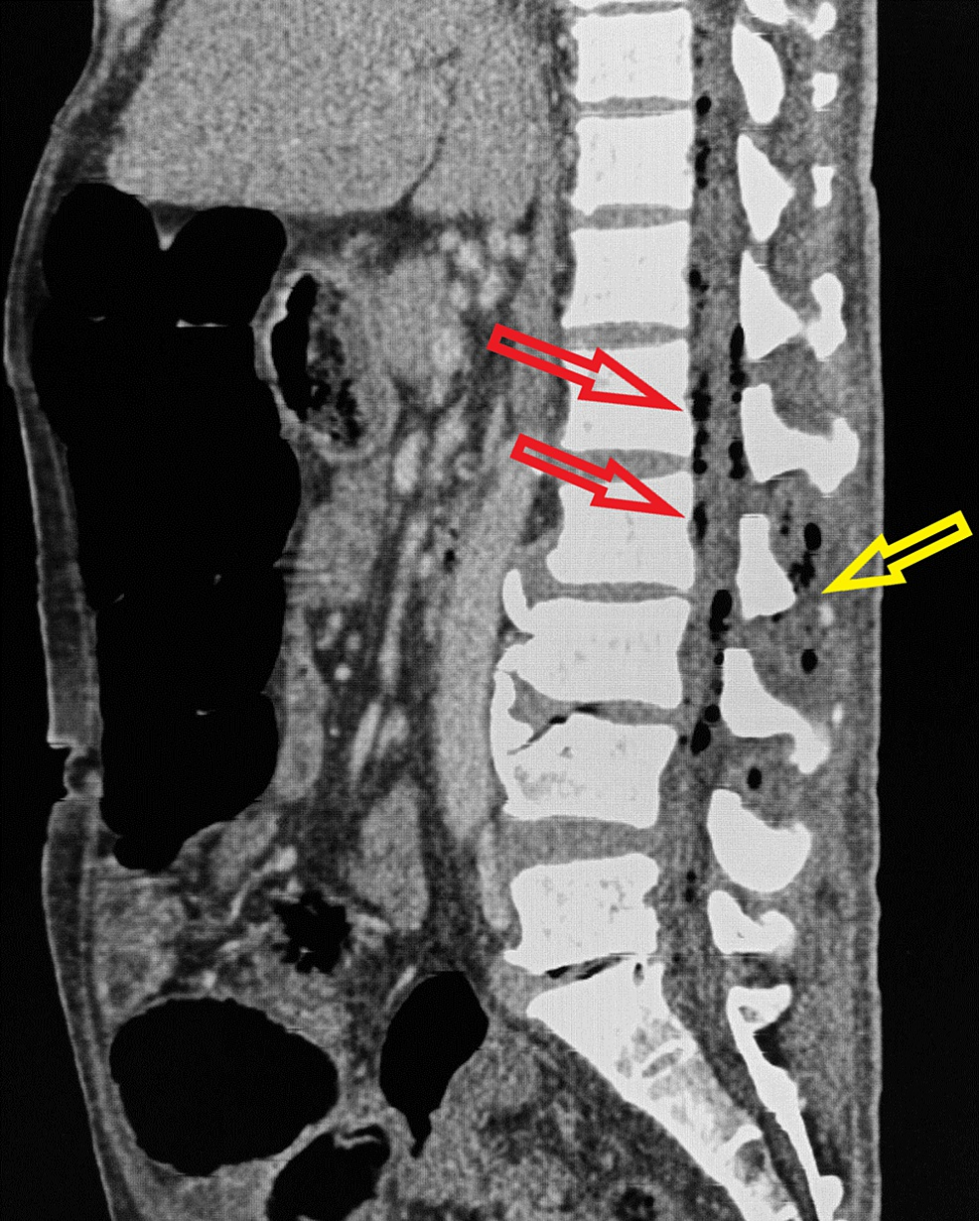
Sagittal contrast-enhanced CT image Show air pockets in the intraspinal epidural space (epidural emphysema) (red arrows) and air pockets in the lower paraspinal muscle on both sides (paraspinal abscess) (yellow arrow).
